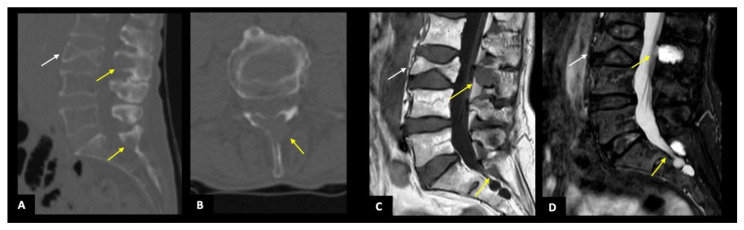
A 67-year-old man with a focal infiltration pattern (A) MPR image in a sagittal view showing some typical lytic lesions localized in the spinous processes of L3 and S1 (yellow arrows) and a vertebral fracture of L3 (white arrow). The sagittal plane allows a proper assessment of the regular alignment of posterior vertebral bodies. (B) CT axial section of L3 showing a lytic lesion with focal cortical interruption of the spinous process. The same bone lesions in the spinous processes of L3 and S1 are visible in images (C) as areas of hypointensity in the T1-weighted sequence of the spine (yellow arrows) and (D) as areas of hyperintensity in STIR-weighted sequence of the spine (yellow arrows). The vertebral collapse of L3 has confirmed both in (C,D) images (white arrow) but better dated as non-recent (no evidence of edema on STIR sequence).
